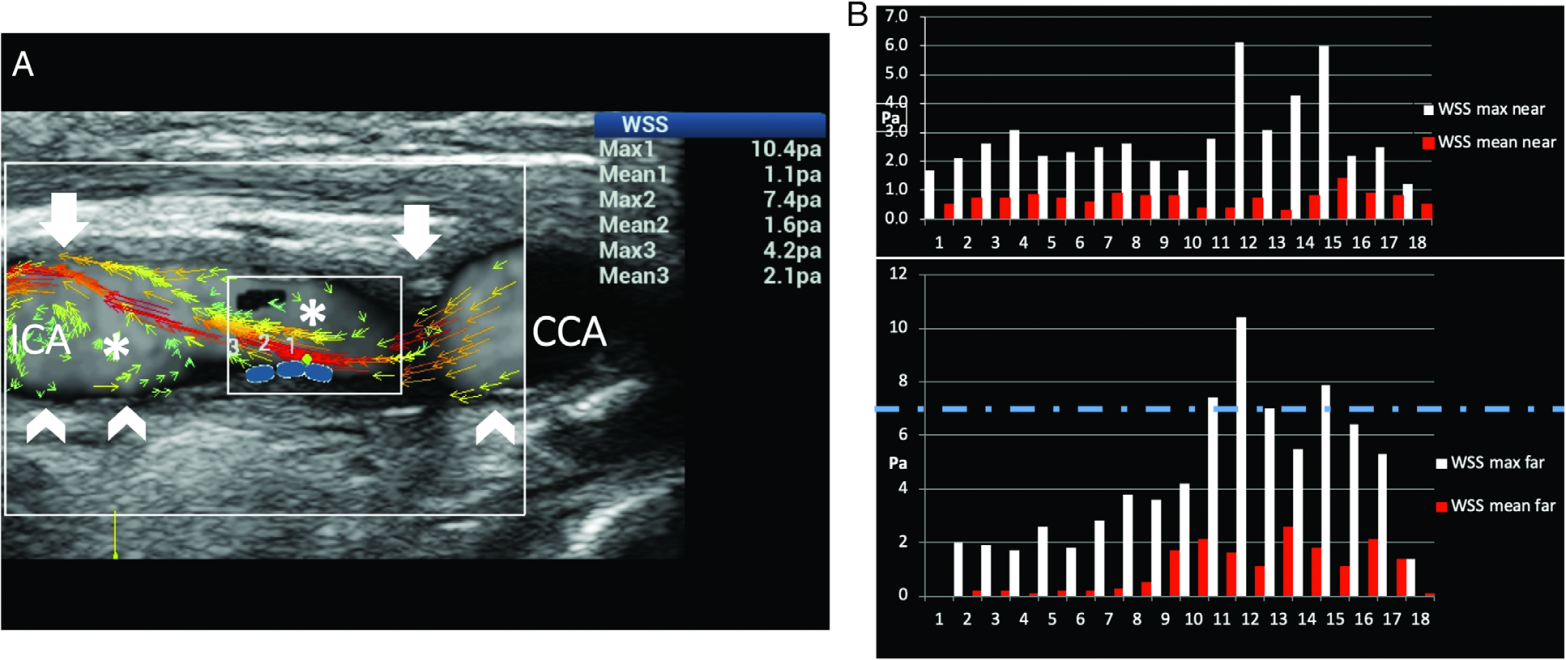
Moderate carotid stenosis. A, Ultrasound vector flow imaging shows high‐velocity red vectors at the level of and distal to the stenosis (arrows), associated with turbulent flow in the poststenotic area (asterisk). B, The graphs obtained by measuring WSS point by point along the near and far vessel walls show a high WSS maximum value of 10.4 Pa (dashed line corresponds to the maximum normal value) in the poststenotic area of the far wall (blue dots on A). Abnormal values low mean WSS values (0.1–0.3 Pa) were detected at the proximal edge of the plaque (0.1 Pa) and distally (0.1–0.3 Pa) on the far wall (arrowheads on Figure A). CCA indicates common carotid artery; and ICA, internal carotid artery.
